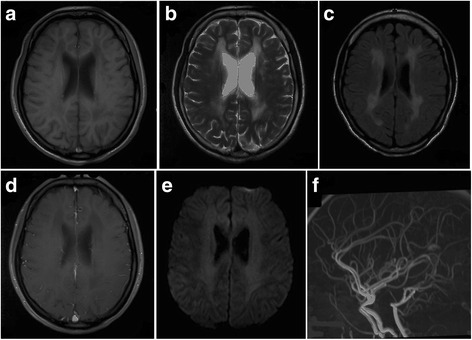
Axial Brain MRI showed low T1-weighted signals (a), high T2-weighted signals (b), and hyperintensity in FLAIR image(c) around the periventricular area. MRI with contrast enhancement was normal (d). DWI revealed slight hyperintensity (e). MRA were normal (f)
